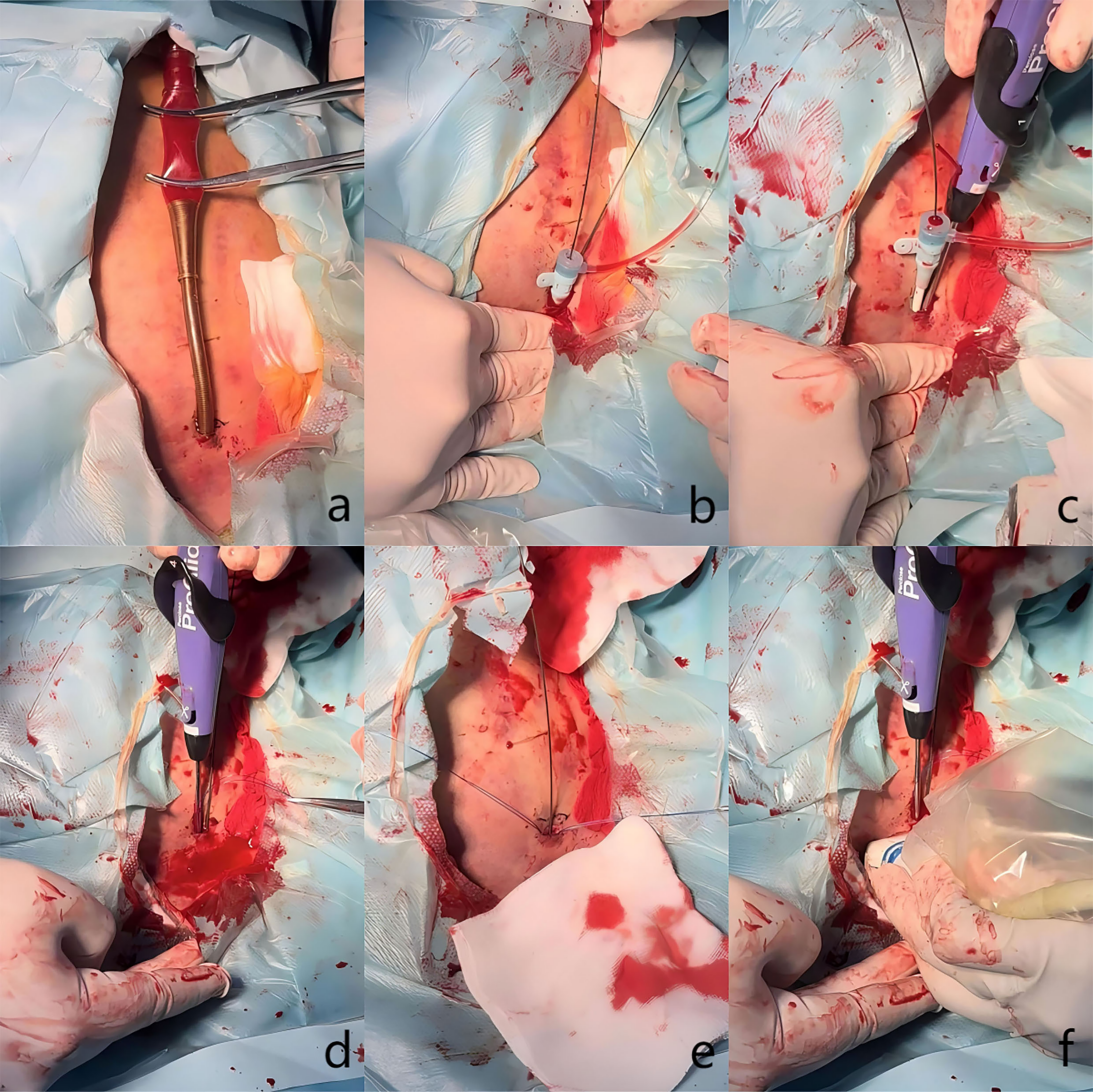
Clinical details of the technique. (a) The arterial cannula was clamped with two forceps. (b) Two guidewires were advanced and an 8 Fr sheath was introduced. (c) The first Proglide device was deployed to control bleeding. (d) The second Proglide device was deployed to control bleeding. (e) Hemostasis was achieved. (f) Proper position of the device's foot pedal was confirmed through ultrasound examination.
